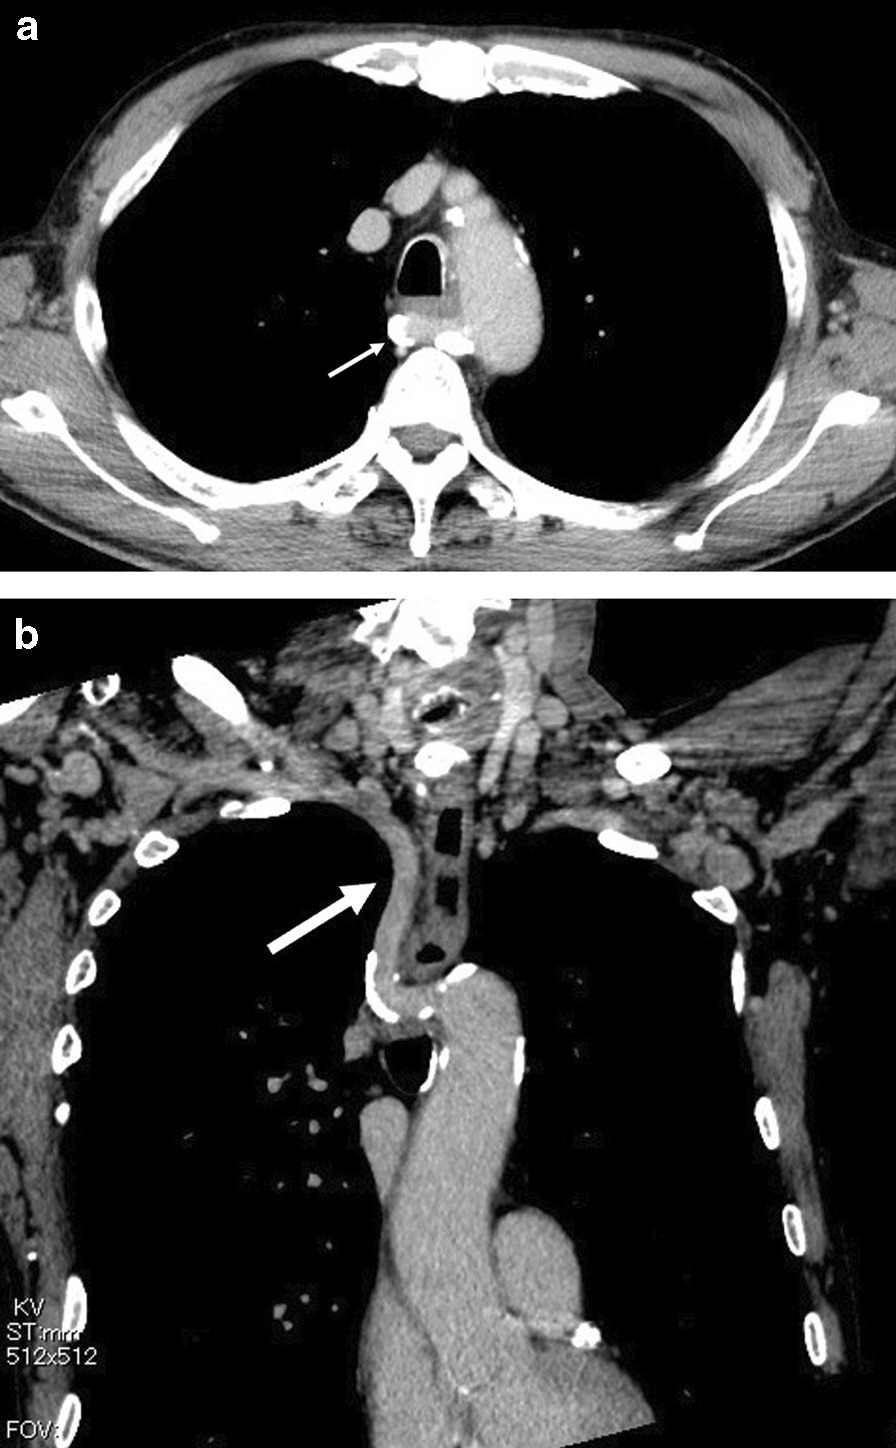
Computed tomographic image showing the aberrant right subclavian artery (arrow). a Transverse plane. b Coronal plane
